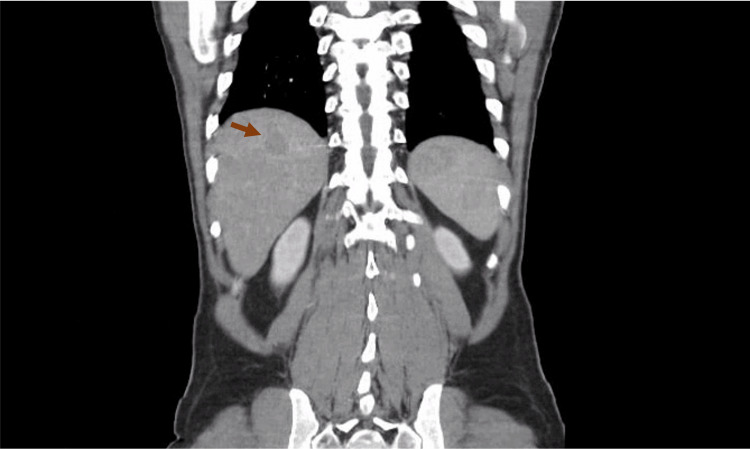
Abdominal CT Scan Hepatosplenomegaly with hypodense nodular lesions (arrow) in the liver and prominent lymph nodes in the celiac-mesenteric region.
